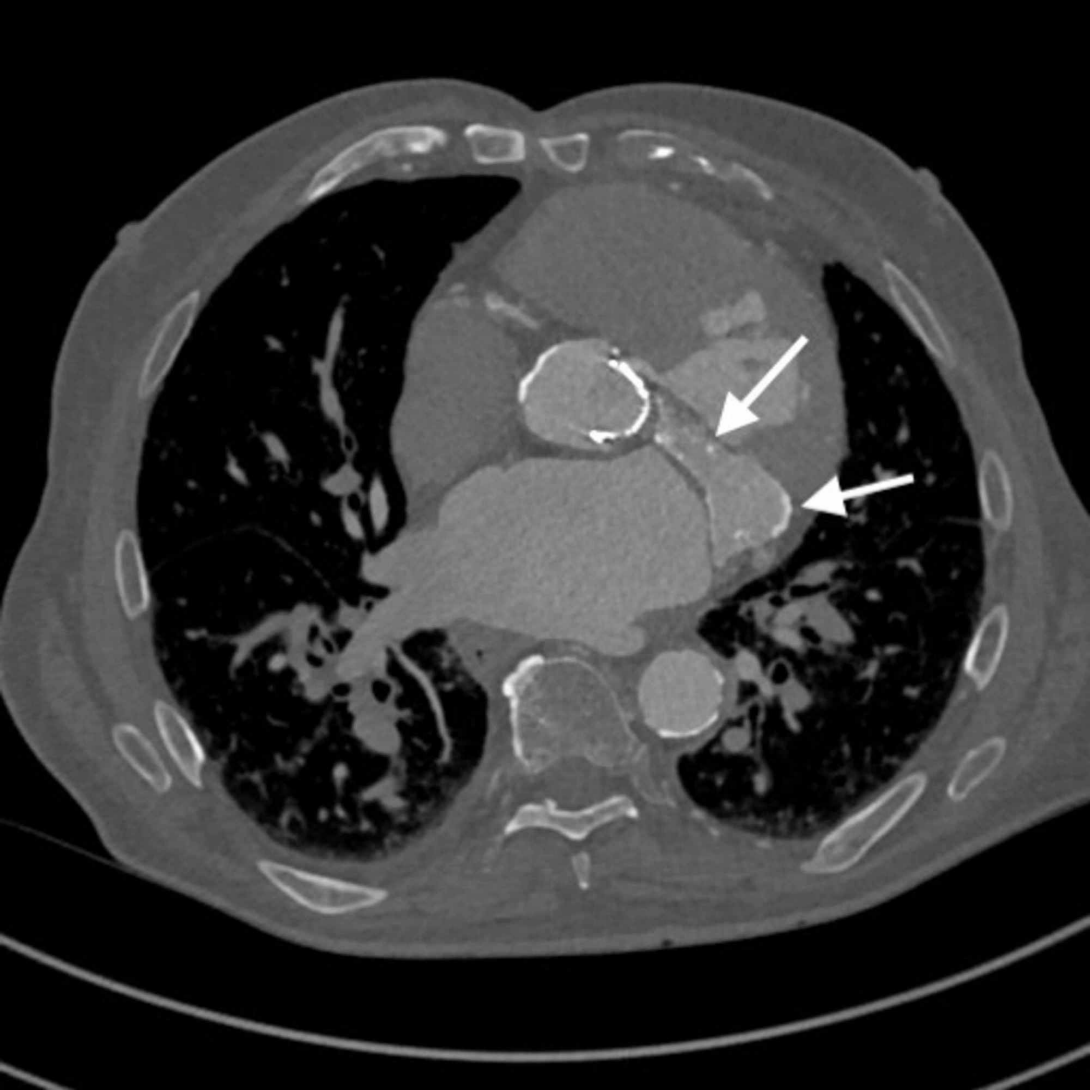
Axial contrast-enhanced CT superiorly to Figure 2. Frank enlargement and internal necrosis with circumferential calcification throughout the mitral valve annulus is better visualized.
